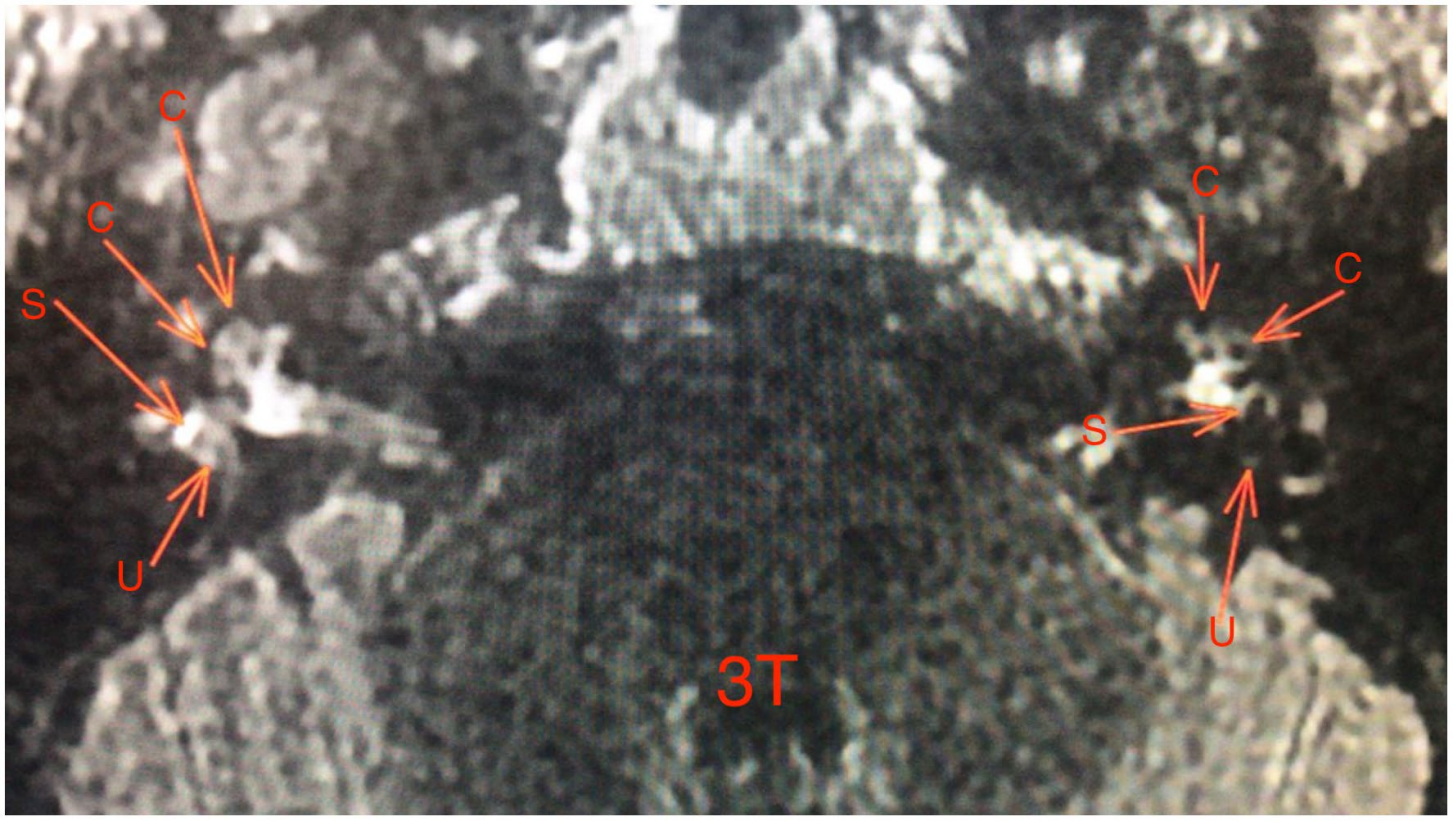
3 T MRI axial scan, sequence 3D-FLAIR, right ear with endolinphatic hydrops in cochlea and vestibule grade 0 and left ear with grade 2 of Barath in cochlea and vestibule.
